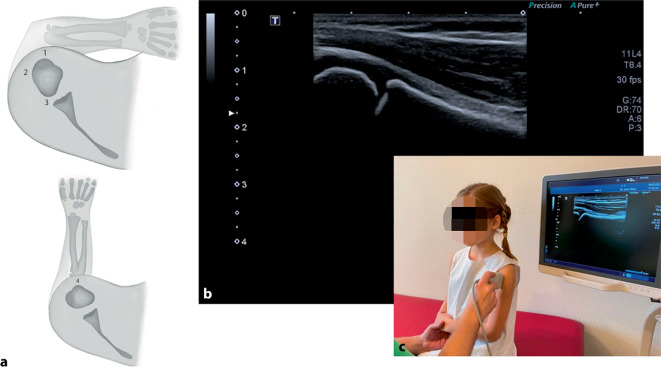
a Sonographische Standardschnitte am proximalen Humerus im Piktogramm (*1* Anterior, *2* Lateral, *3* Posterior, *4* Medial). b Darstellung des proximalen Humerus (*links* die Epiphyse, *nach rechts auslaufend* der Humerusschaft). c Positionierung des Kindes zur schmerzarmen Untersuchung
